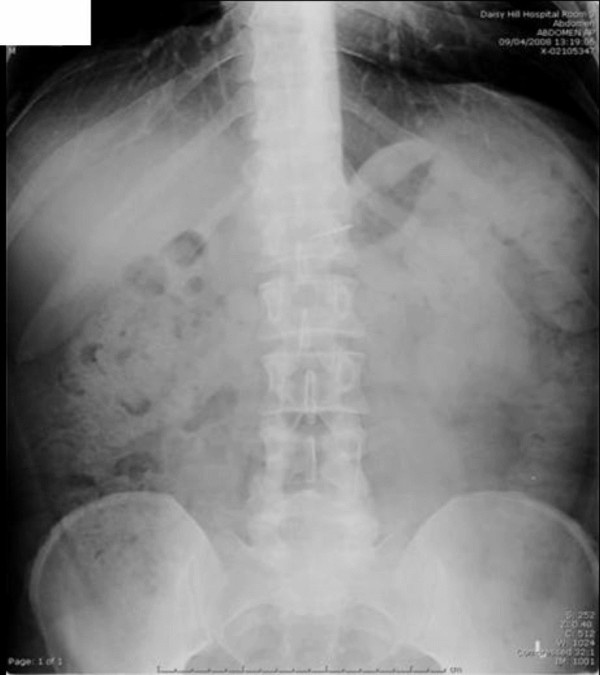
Abdominal X-ray (AXR) demonstrating root canal file at the level of the L1 vertebra.
